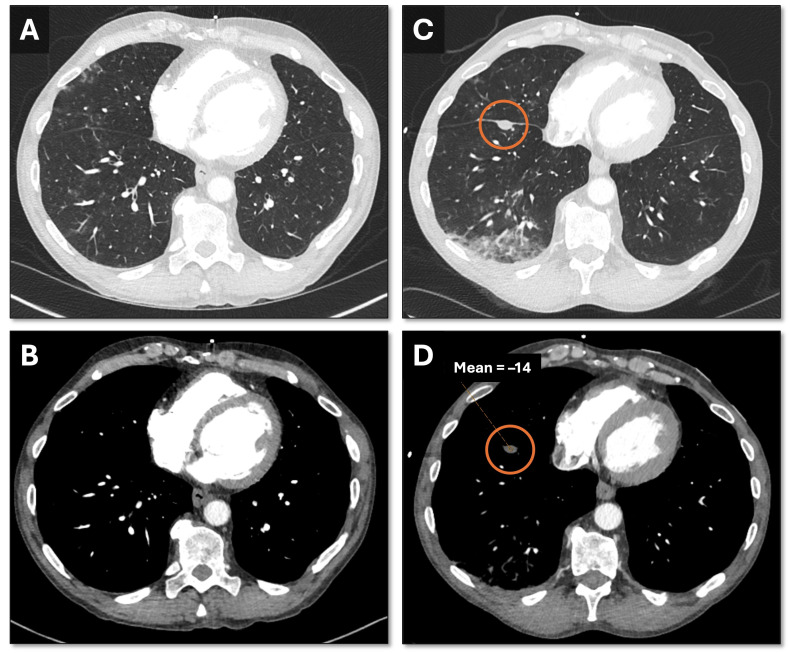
Loculated pleural effusion in a patient with a history of heart failure. An example of a benign mimic of juxtapleural nodules. Axial CT images prior to effusion in (A) lung and (B) mediastinal windows and after effusion in (C) lung and (D) mediastinal windows. The red circle highlights the loculated pleural effusion.The mean Hounsfield attenuation of the fluid is −14, based on delineated region of interest.
